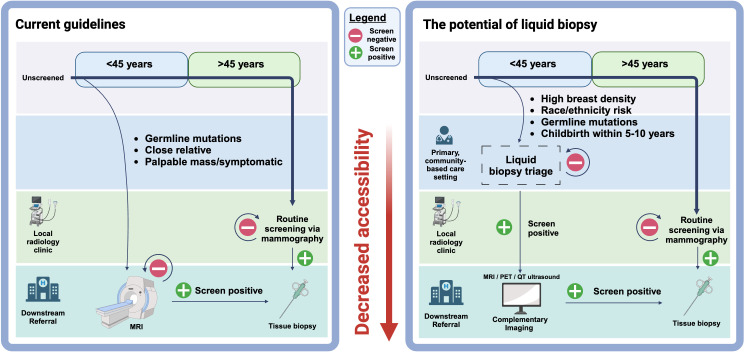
The potential of liquid biopsy to improve breast cancer screening accessibility through primary, community-based care. The current breast cancer screening guidelines do not have recommendations for several at-risk populations due to lack of diagnostic technology. Implementation of liquid biopsy early detection tests may allow for breast cancer screening to be accessed through a primary physician instead of a specialized clinic. This would address barriers of distance to facility, travel time and transportation. There is still a need for improvement of imaging modalities such as MRI, PET and QT ultrasound to be used complementary to liquid biopsy.
